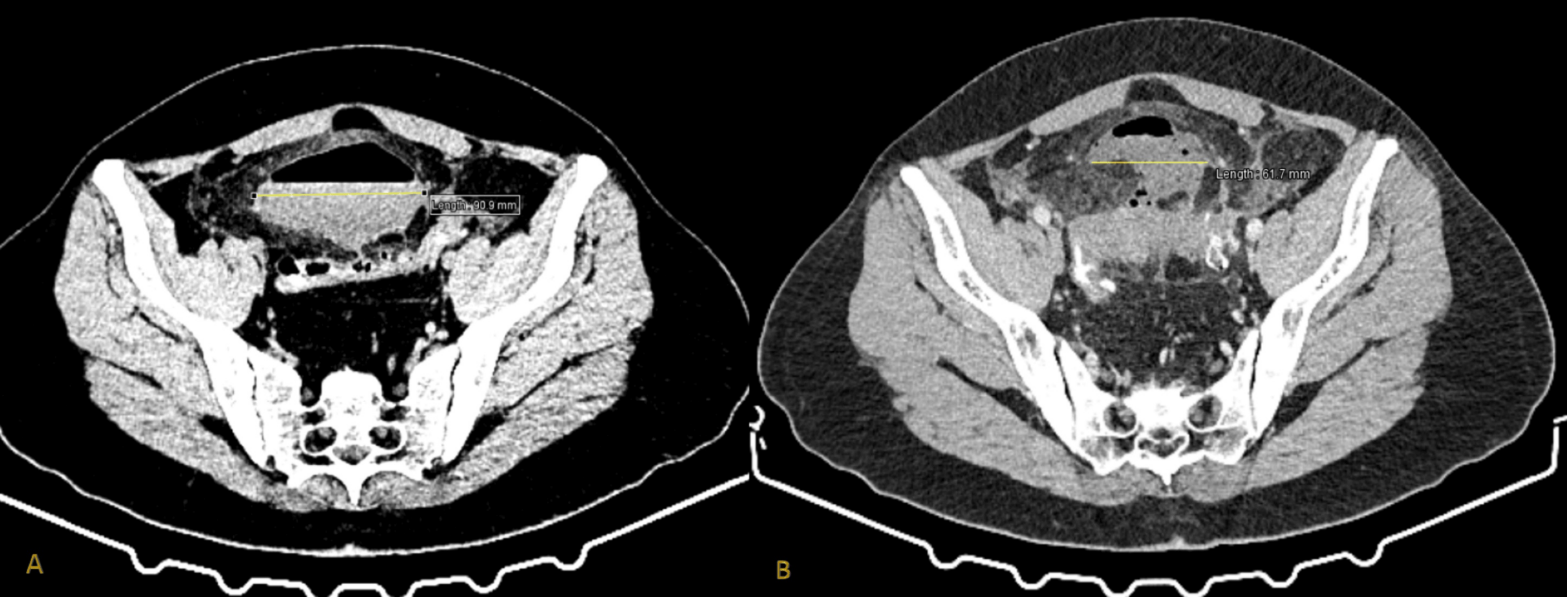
Clinical example of a Hinchey ΙΙ case and its management (A) Hinchey II acute colonic diverticulitis with pelvic abscess: Pelvic abscess measuring 9.09 cm in its maximal diameter in a young male patient who had late presentation (seven days after the onset of LLQ pain) to the emergency department. Due to the localization of the abscess, the age of the patient, and his stable condition, the patient was referred for percutaneous drainage to the gastroenterology department of another hospital, where 450 ml of purulent material was initially aspirated. (B) Post-drainage deterioration and surgical intervention: Due to the localization of the abscess, the age of the patient, and his stable condition, the patient was referred for a second percutaneous drainage of the abscess, which deteriorated to 6.17 cm in length in a new CT scan. After two incomplete drainage attempts, the patient was consequently led to the OR for Hartmann’s procedure. LLQ: Left lower quadrant.
